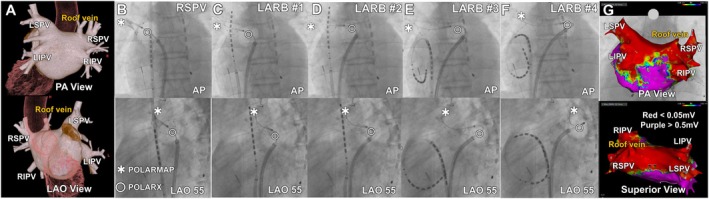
Preoperative computed tomography (CT) and intraoperative fluoroscopic images of the procedure. (A) Pre‐procedural CT demonstrated the roof vein, along with the left superior, left inferior, right superior, and right inferior pulmonary veins. (B) The POLARMAP catheter was positioned at the RSPV, and a 180‐s freeze achieved isolation. Phrenic pacing was performed via a duodecapolar catheter in the right subclavian vein to prevent phrenic injury. (C) The first cryoapplication targeted the left atrial roof, with the POLARMAP catheter advanced deeply into the upper RSPV and a 180‐s freeze delivered using the raise‐up technique. (D, E) The second (D) and third (E) cryoapplications targeted the left atrial roof, with the POLARMAP catheter anchored in the upper RSPV and sequential overlapping freezes delivered using the same rotational and sheath‐manipulation technique. (F) The fluoroscopic images show the fourth cryoapplication directed at the left atrial roof. During this freeze, the cryoballoon lost stable anchoring and collapsed toward the LSPV. Repositioning the POLARMAP catheter into the roof vein (RV) allowed successful completion of the freeze. (G) Post‐mapping using the CARTO3 system (Biosense Webster) confirmed durable pulmonary vein isolation and complete LARB creation. AP, anterior to posterior; PA, posterior to anterior; LAO, left anterior oblique; RSPV, right superior pulmonary vein; RIPV, right inferior pulmonary vein; LSPV, left superior pulmonary vein; LIPV, left inferior pulmonary vein; LARB, left atrial roof block.
